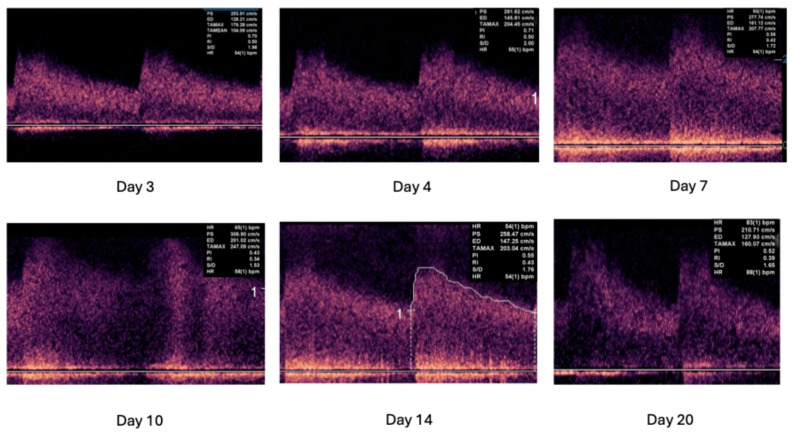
Transcranial Doppler registrations during development and resolution of cerebral vasospasm.
